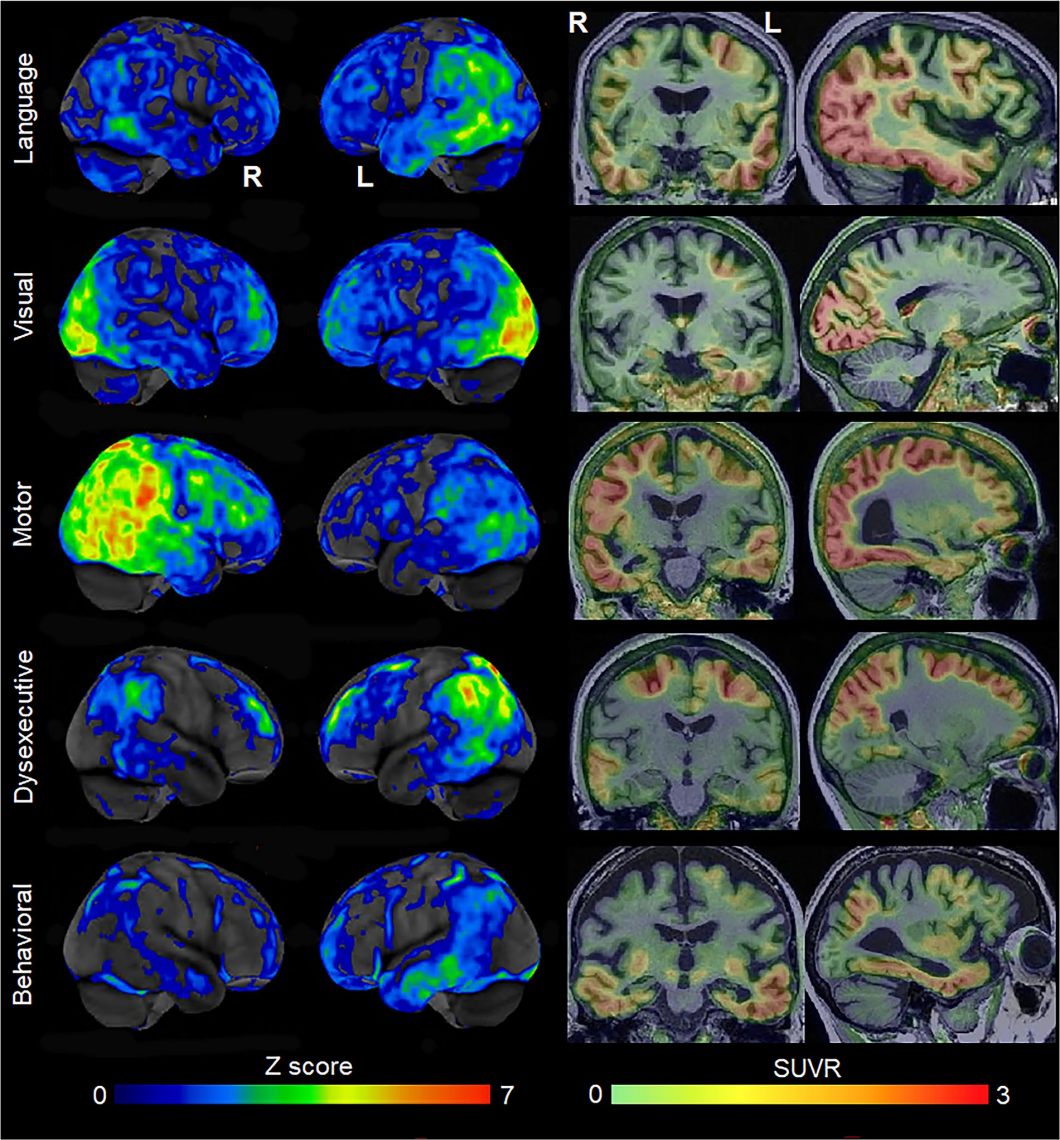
Patterns of FDG-PET hypometabolism and flortaucipir PET uptake in individual patients with different variants of atypical AD. The FDG-PET images are shown as CortexID Z score images of hypometabolism compared to age-matched controls. The flortaucipir PET images are shown as standardized uptake value ratio (SUVR) images referenced to the cerebellar crus grey matter. The language variant of AD shows left temporoparietal hypometabolism and flortaucipir uptake; the visual variant shows abnormalities predominantly in the occipital lobe; the motor variant shows abnormalities in the posterior temporal, parietal and frontal lobes, predominantly on the right; the dysexecutive variant shows left-sided abnormalities predominantly in the superior parietal and frontal lobes; the behavioral variant showed abnormalities in the temporal lobes, with milder changes in the parietal and frontal lobe.
